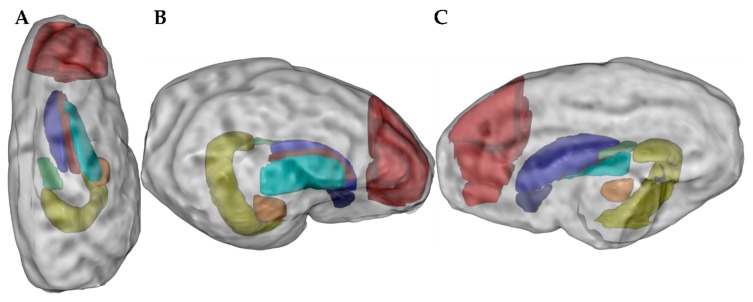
Regions of interest (ROIs) after magnetic resonance imaging presented in top view (A), lateral view (B), and medial view (C), respectively. Measured ROIs: nucleus accumbens (dark blue), caudate nucleus (blue), lentiform nucleus (cyan), fornix (green), hippocampus (yellow), amygdala (orange), prefrontal cortex (red), and internal capsule (burgundy).
